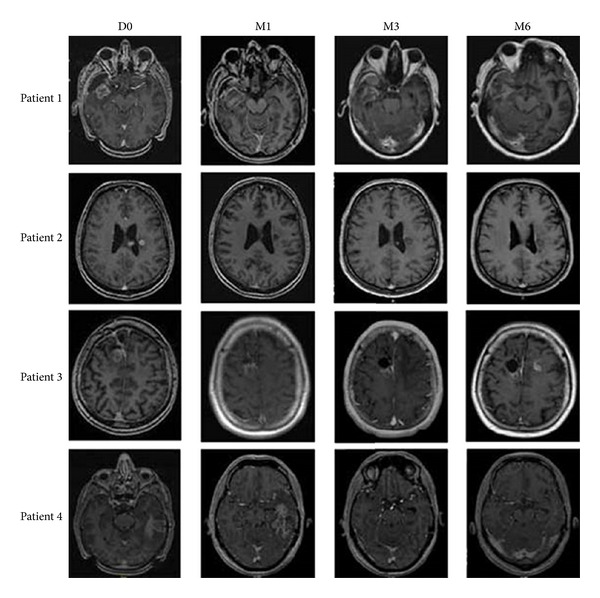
MR imaging of four patients with recurrent glioblastoma, following LITT on the day of treatment (D0), one month following (M1), three months following (3 M), and six months following (M6). Recurrence was observed in all patients, with a mean progression-free survival time of 37 days. Though LITT is suited for addressing many tissue types and tumor shapes/sizes, GBM masses are often diffuse in brain tissue. In order to discriminate between healthy and diseased tissue, highly specific therapies are desirable. Though more developed surgical methods may help in an immediate sense, reliance on simple hyperthermia outside the zones of ablative heat may not be sufficient for addressing microtumors seen in GBM.
